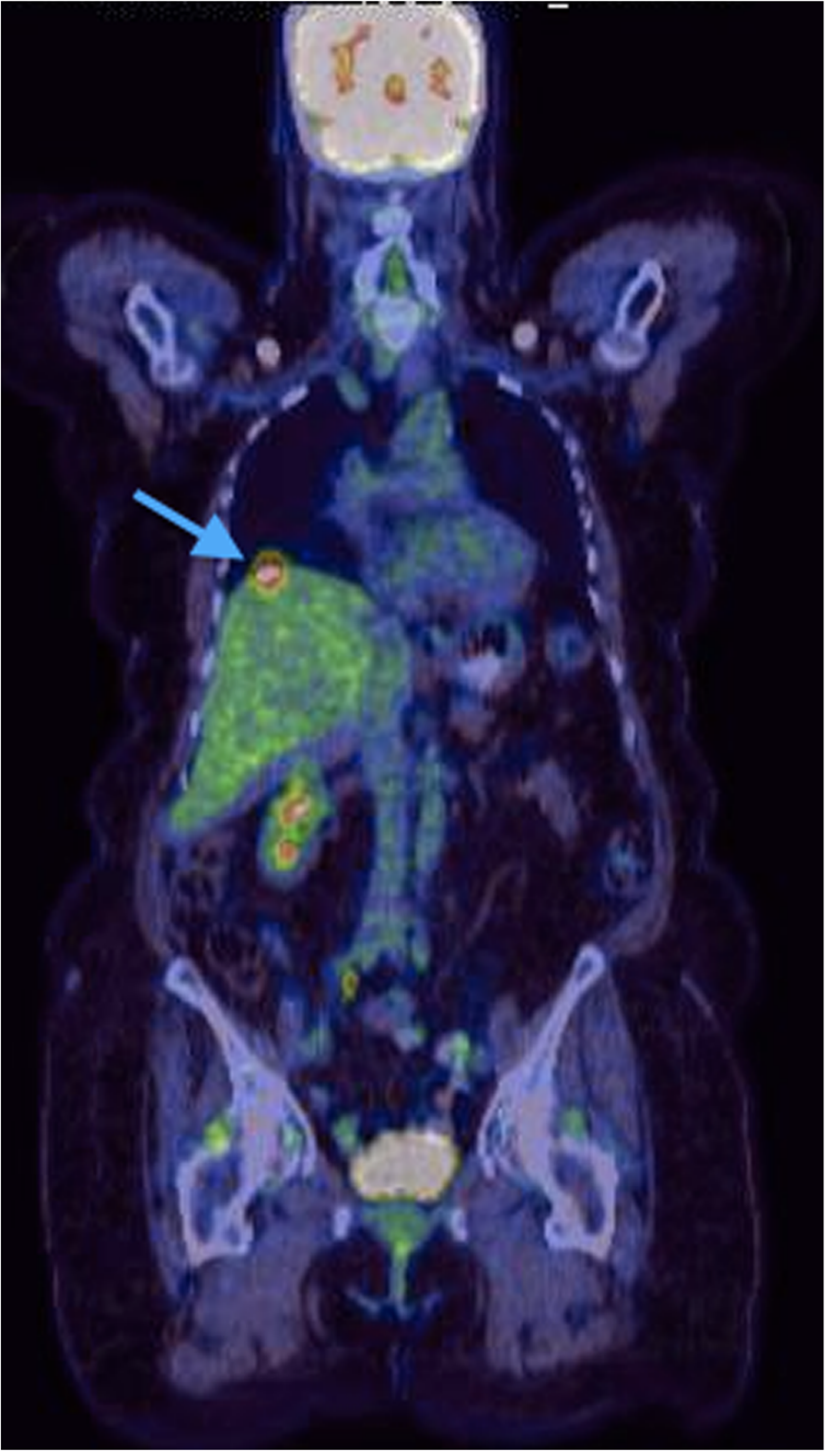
Follow-up PET CT showing evidence of recurrent liver disease localized to the liver (4 months post-redo liver resection): two focal liver uptakes (at the liver dome and in the surgical bed) consistent with recurrence
